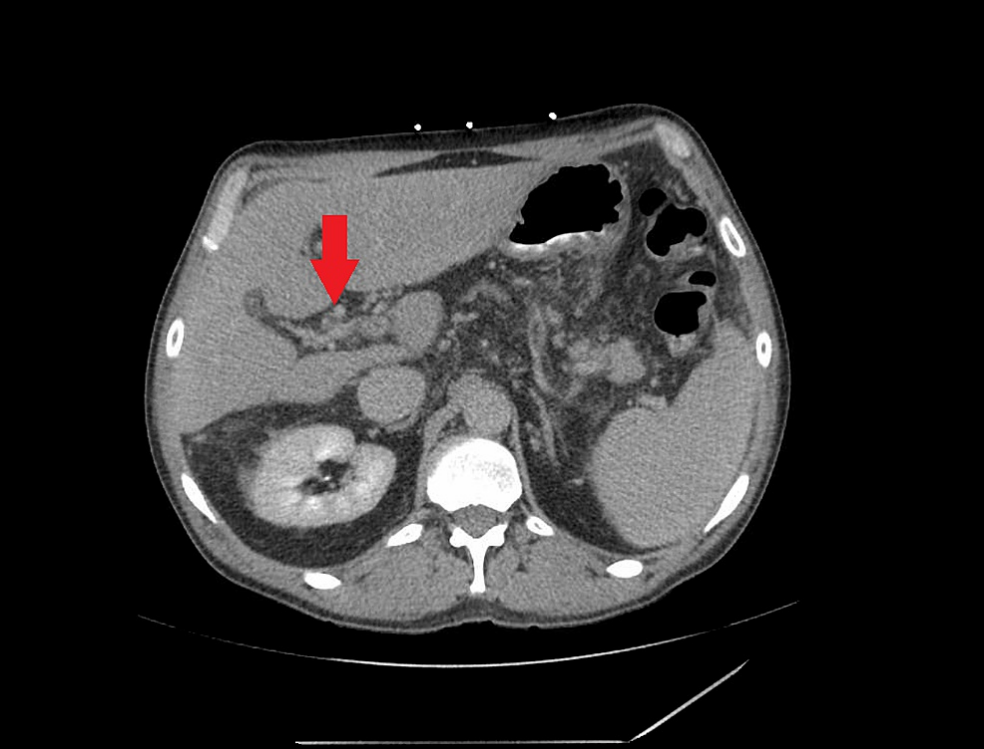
CT scan of the abdomen with IV contrast. Arrowhead demonstrates the cavernous transformation of the portal vein.
